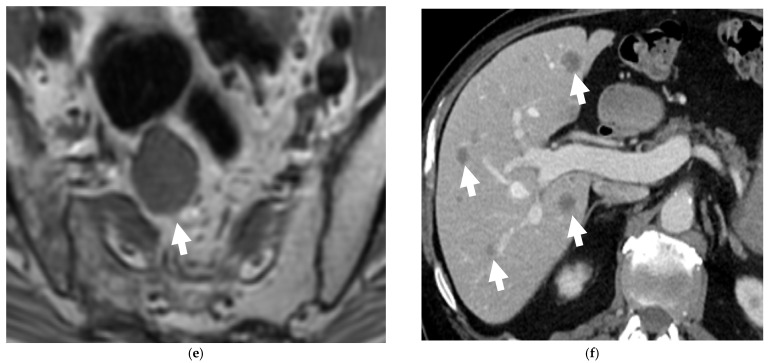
A 60-year-old male with Gleason 9 prostate adenocarcinoma at diagnosis, with later neuroendocrine differentiation after 1 year while on androgen deprivation therapy, with metastatic neuroendocrine prostate cancer. Axial ADC map of the MRI prostate (a) shows hypointense right prostatic nodule (with restricted diffusion), consistent with prostatic cancer (long arrow). There is a large mass along the right posterolateral aspect of the prostate, consistent with extraprostatic extension (short arrow). Axial T1W fast spin echo (FSE) image (b) shows bony metastasis involving the left posterior acetabulum. Patients’ serum PSA decreased following androgen deprivation therapy. Follow up MRI after 7 months revealed improving (smaller) extraprostatic component of the prostatic mass and left acetabular bony metastasis with relatively unchanged size of the prostatic neoplasm on the apparent diffusion coefficient (ADC) map (c) and axial T1W FSE (d) images. However, newly enlarged right presacral node (arrow) seen on T1W FSE (e) of follow-up MRI pelvis and multiple new liver lesions (arrows) seen on CECT (f) at 10 months and 18 months, respectively, after the diagnosis, were biopsied and proven to be metastatic adenocarcinoma with neuroendocrine differentiation.
